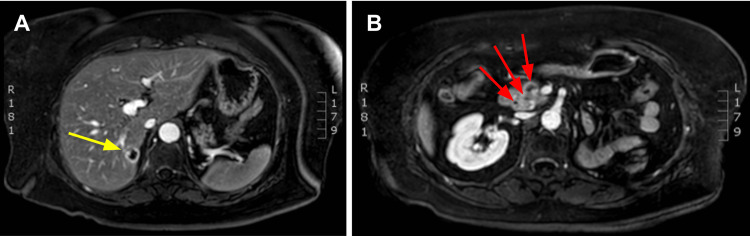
Contrast-enhanced abdominal MRI. (A) Focal liver lesion of 1.7 cm in segment 6th of the right hepatic lobe (yellow arrow). (B) Multiple nodular lesions in the pancreas (red arrows).
